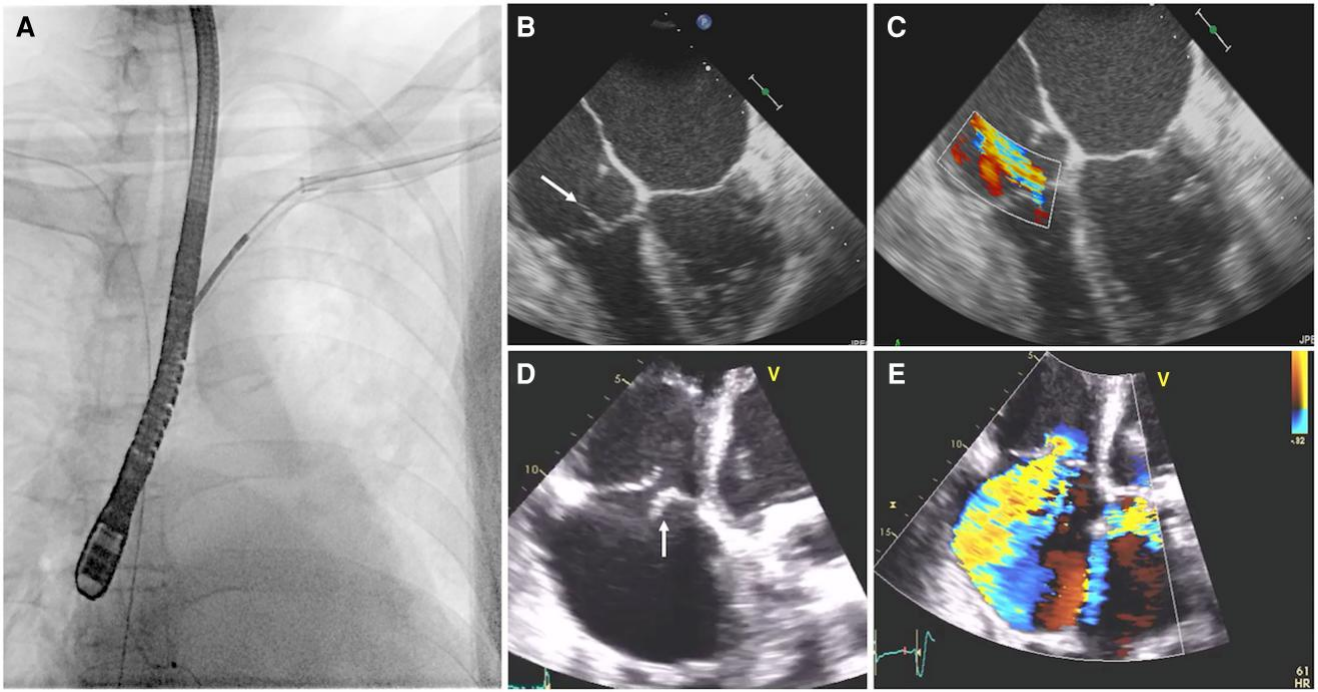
Echocardiography evaluation following TLE showing severe TR and flail tricuspid leaflet (white arrow). (*A*) Transvenous rotational mechanical lead extraction. (*B* and *C*) TEE evaluation immediately after the procedure. (*D* and *E*) TTE evaluation after the procedure confirming severe TR and flail tricuspid leaflet. TEE, transoesophageal echocardiography; TTE, transthoracic echocardiography.
